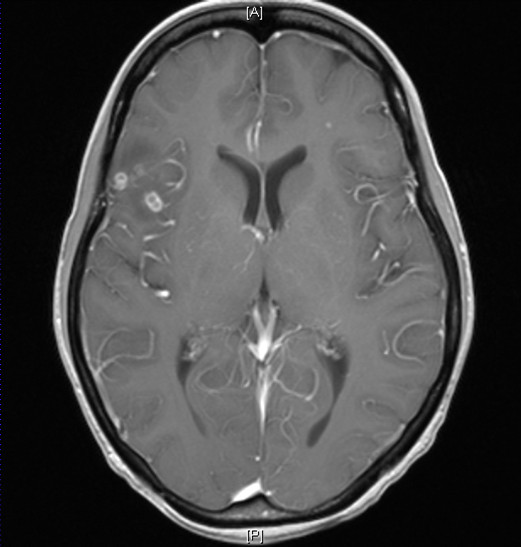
MRI brain scan performed mid-treatment. The MRI brain scan performed shortly after readmission with acute neurological symptoms, demonstrates a number of ring-enhancing lesions with surrounding oedema which were thought likely to be metastases.
